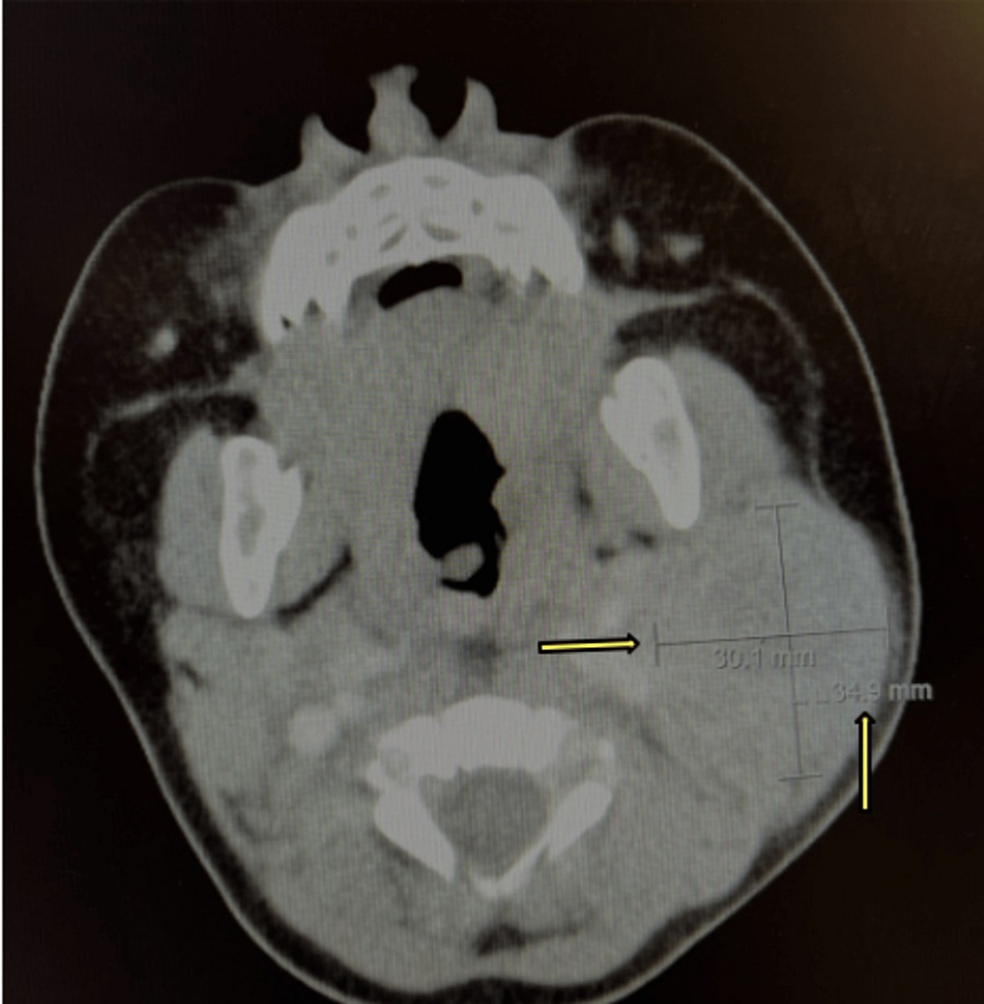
Axial CT with contrast showing heterogeneous enhancement (pointed by yellow arrows)
